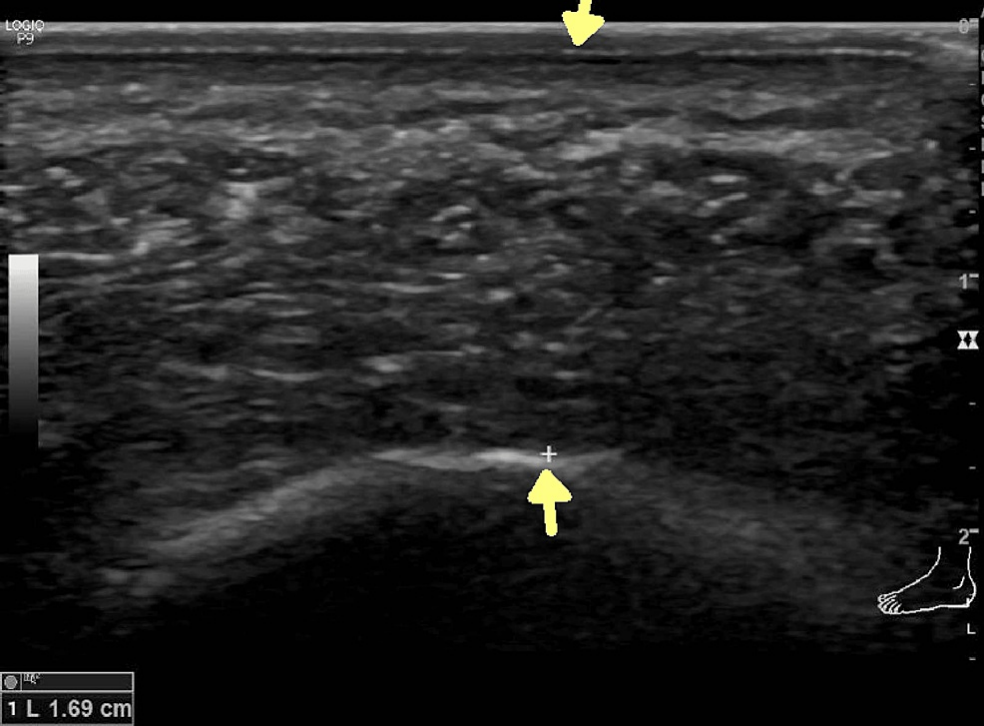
Ultrasonographic picture showing normal heel pad thickness (yellow arrows)
